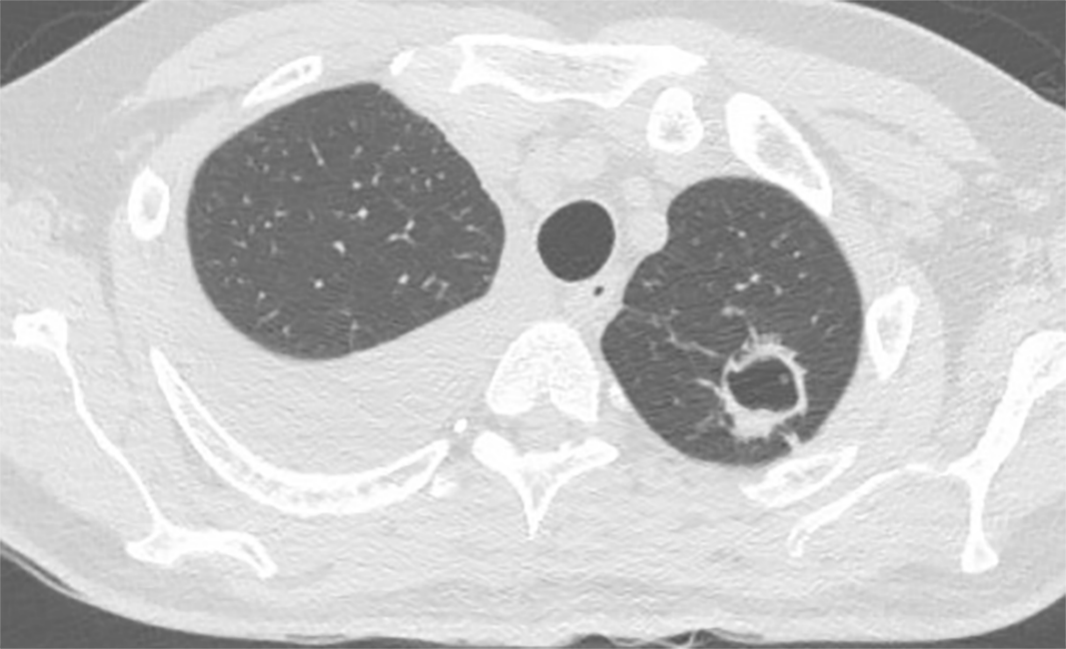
A 48-year-old man with dermatomyositis, currently undergoing combination therapy with leflunomide and methotrexate, presented with chronic dyspnoea. A chest CT scan revealed a right chronic pleural effusion (a rare finding in CPH) with associated thickened pleura and a cavitary lesion in the left upper lobe. He was diagnosed with pulmonary histoplasmosis following positive serology with high titers and confirmation via lung biopsy.
